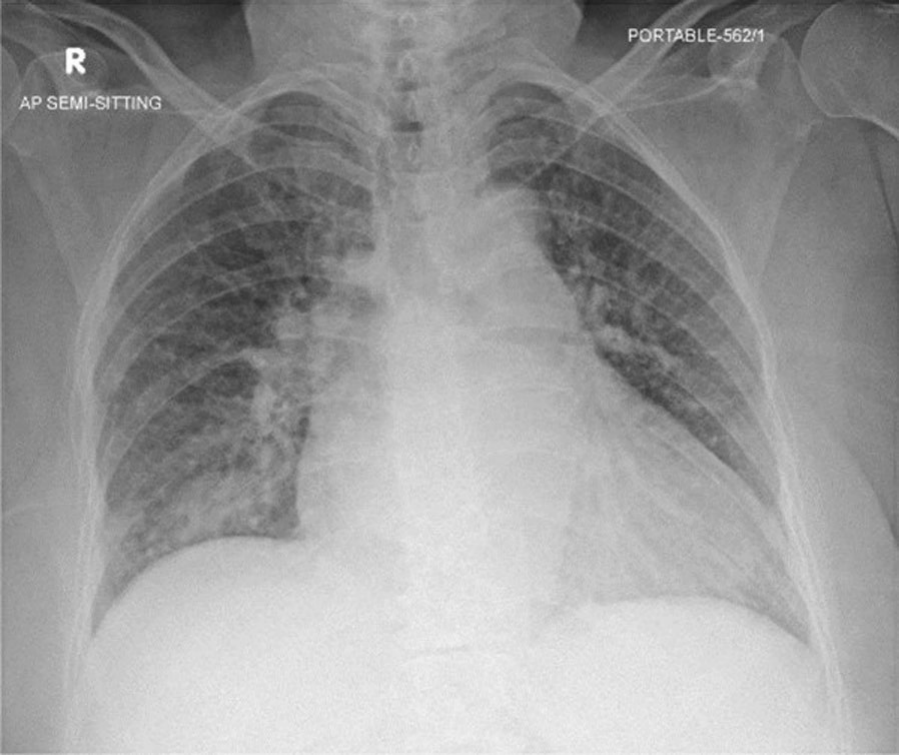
Anteroposterior (AP) chest X-ray on the day of admission
